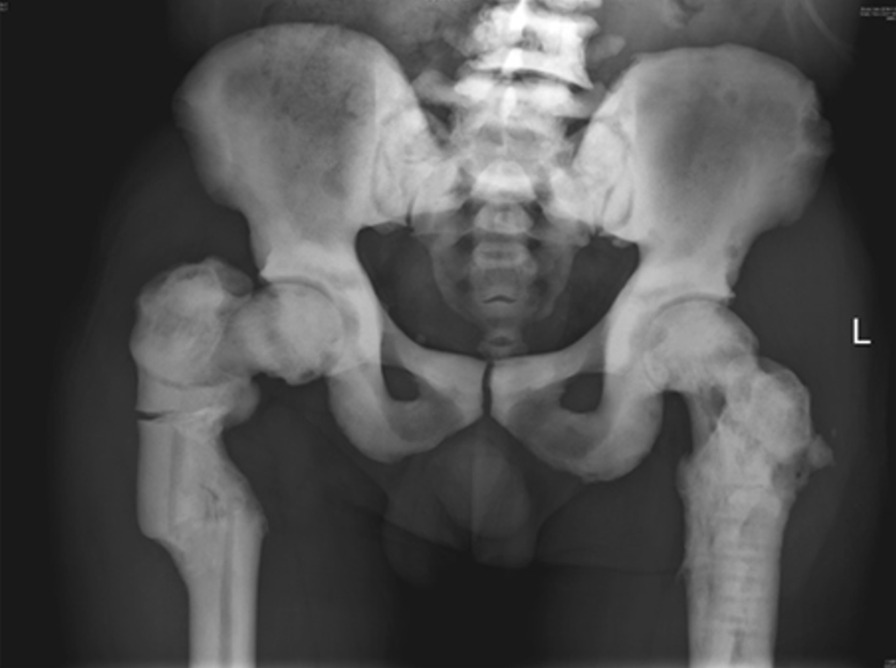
Radiograph taken three days before the first operation
